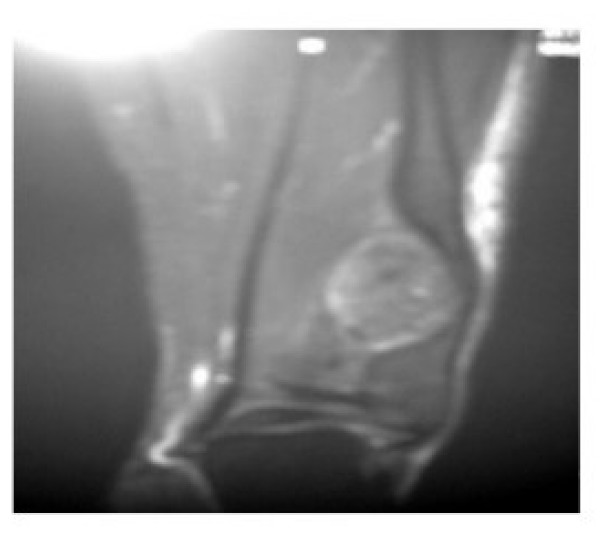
MRI of the ankle showing osteochondroma with an uncalcified cartilaginous cap.
